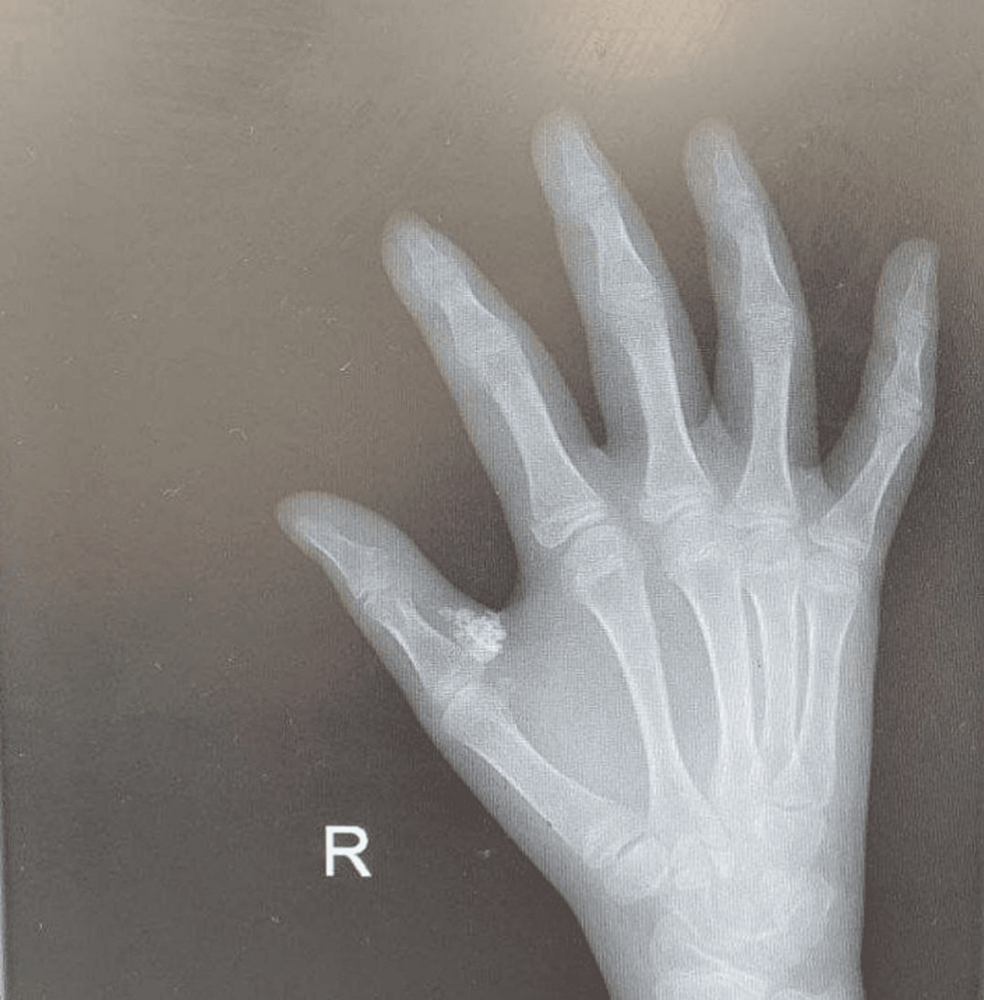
Plain X-ray of the recurrent calcinosis cutis specifically affecting her right thumb's metacarpophalangeal joint
